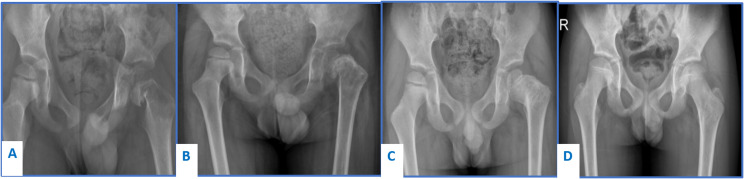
Plain radiograph of the left hip of a 5-year-old boy diagnosed with LCH in the femoral neck managed conservatively. (A) Pathologic fracture of the left femoral neck on top of lytic lesion. (B) Healing of the fracture after 3 months. (C) Remodeling of the femoral neck at 1 year of follow-up. (D) Complete remodeling after 7-year follow-up.
